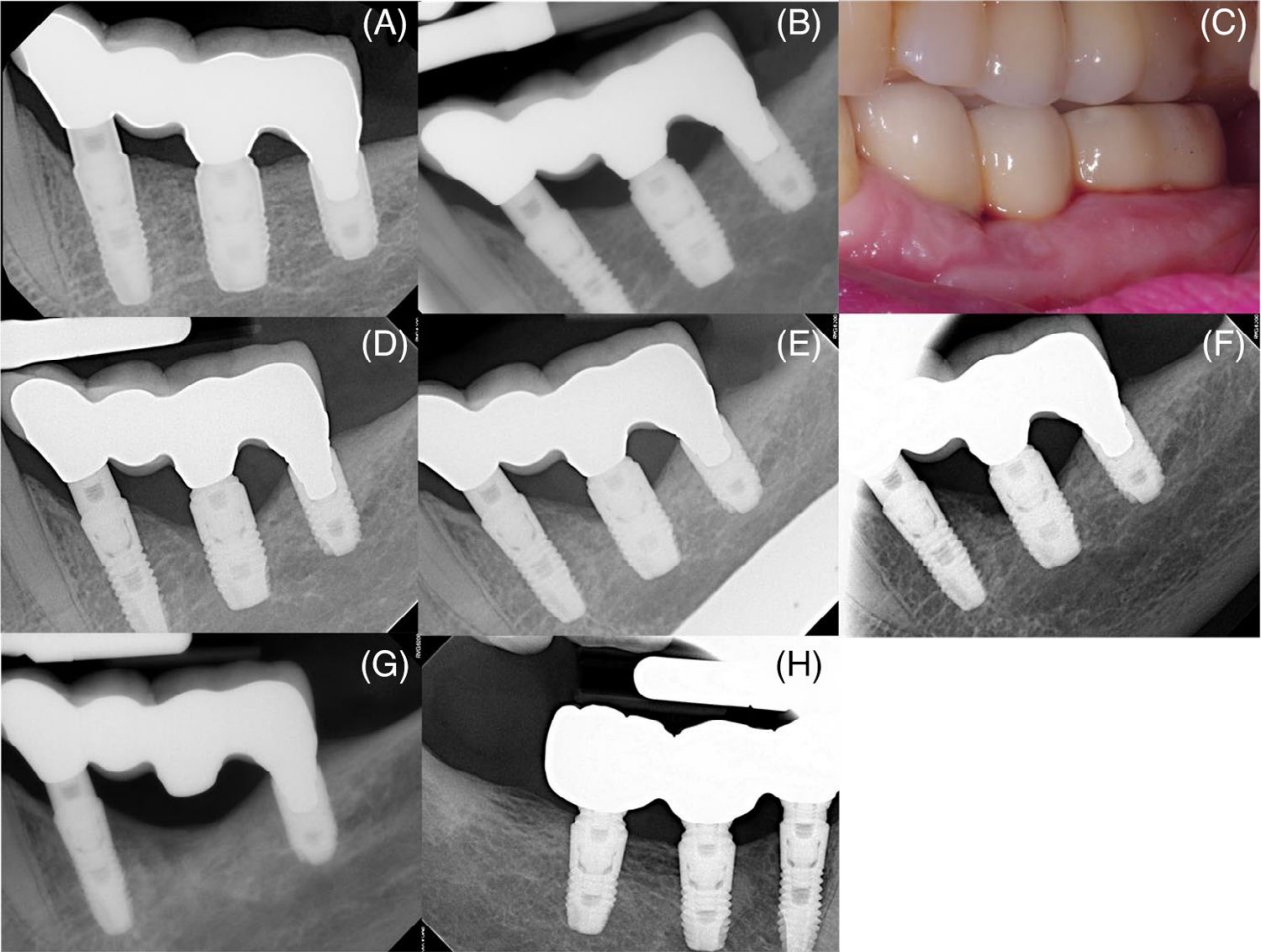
Radiographic series and one clinical photograph for patient 1. (A) A 3‐IS four‐unit FDP was placed in the mandibular left posterior sextant in 2016 (Astra Tech OsseoSpeed TX); (B) early signs of peri‐implant bone loss were suspected in 2018; (C) clinically the implants appeared healthy; after subsequent follow‐up in (D) 2019, (E) 2020, and (F) 2021, the middle implant was determined to be hopeless, was explanted, and the modified FDP re‐inserted; (G) at 3‐years, the modified FDP in the mandibular left posterior sextant and (H) the 3‐IS three‐unit FDP in the mandibular right posterior sextant both appear radiographically stable.
